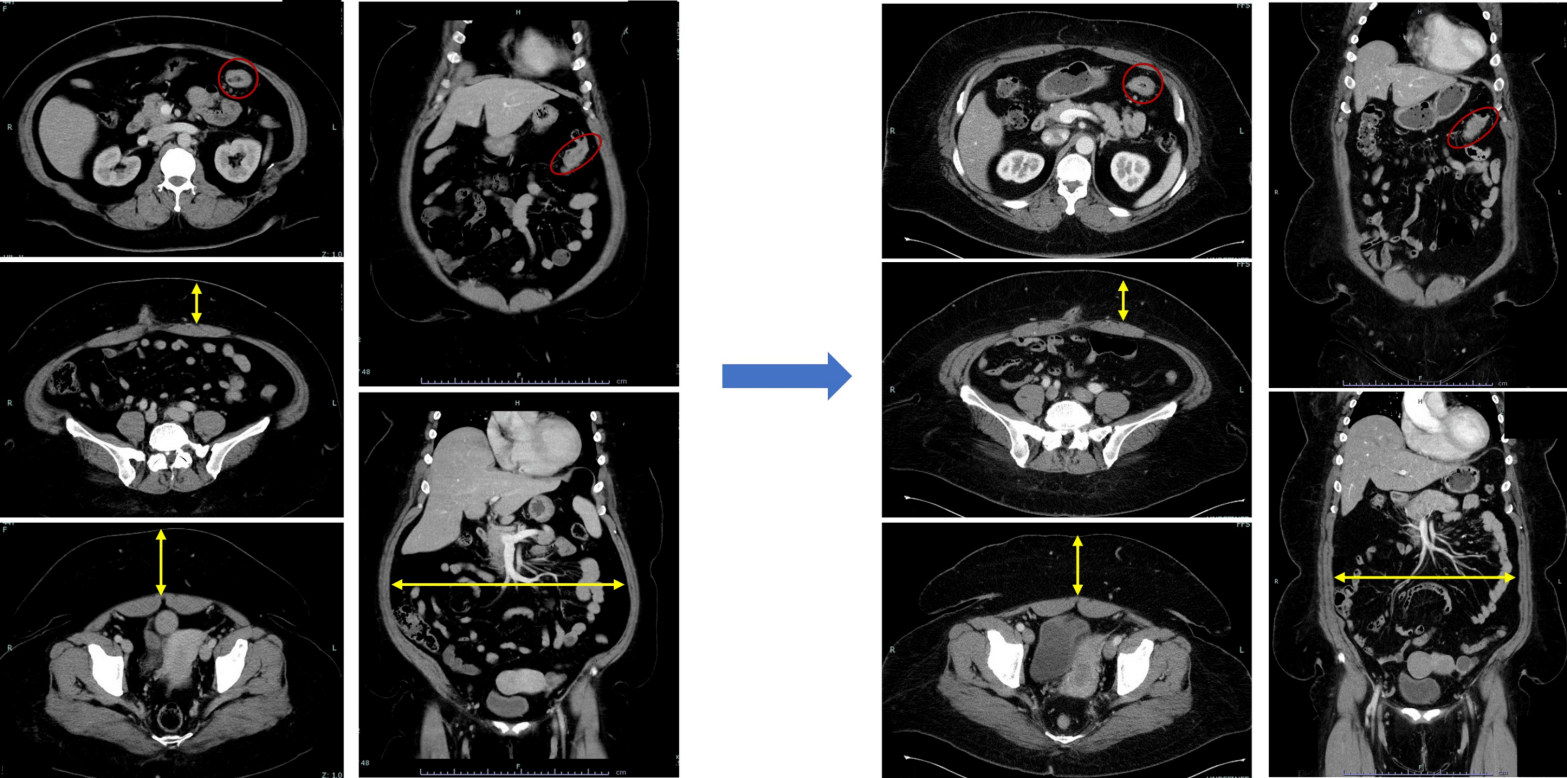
CT before and after weight loss treatment CT shows no obvious change in tumor and lymph node size before and after weight loss treatment (red-lined area), but CT shows a decrease in abdominal circumference and visceral fat (yellow arrows). CT: computed tomography
